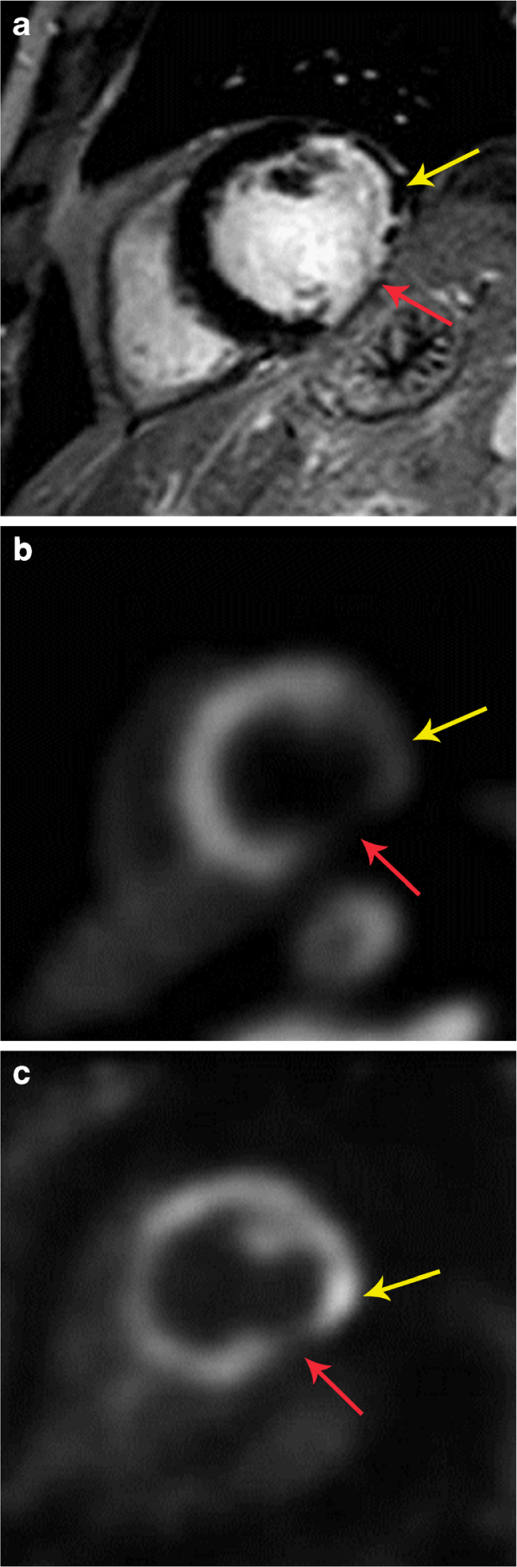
Hibernating myocardium. a 57-year-old male with coronary artery disease, status post multiple prior percutaneous interventions, with symptoms of angina at rest. Short-axis delayed enhancement MRI shows partial thickness scar in the anterolateral segment (yellow arrow) and near-full thickness scar in inferolateral segment (red arrow). b Rb-82 PET rest perfusion image shows perfusion defect in the anterolateral and inferolateral segments (yellow and red arrows, respectively). This was similar on stress images as well. c FDG-PET image shows no uptake in the inferolateral segment (red arrow) indicating non viability, while there is uptake in the anterolateral segment (yellow arrow), indicating hibernating myocardium
